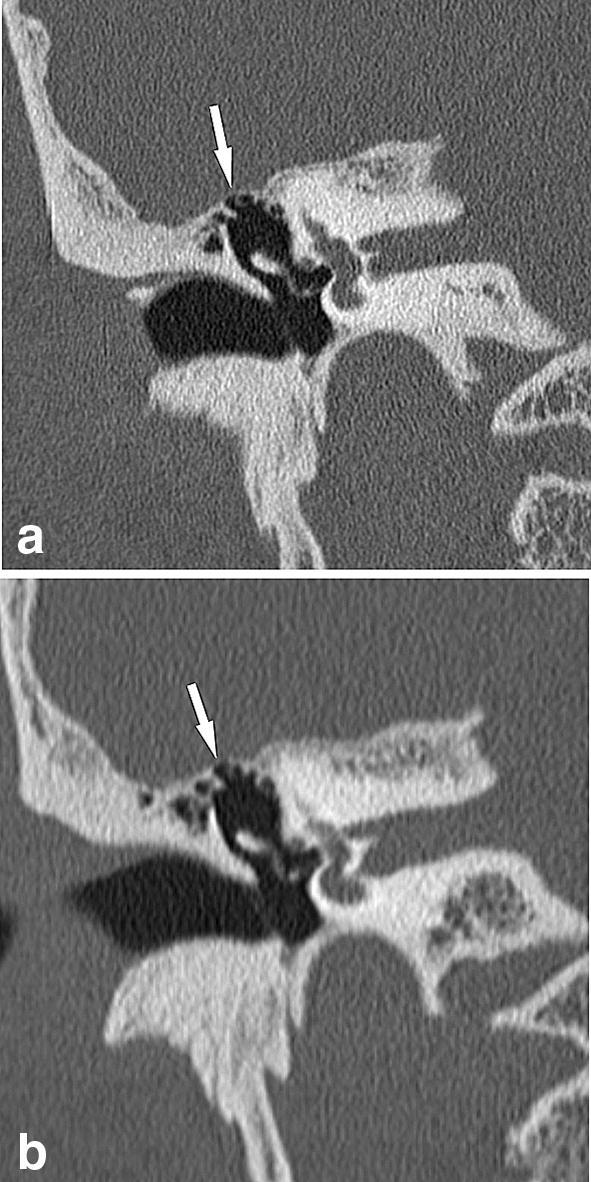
Coronal images of the right temporal bone CT from the same case. Image quality scores for the tympanic tegmen (arrow) are five on UHRCT (a), and three on MDCT (b) by both Reviewers. By both Reviewers, dehiscence in this anatomy was considered to be present on MDCT but not on UHRCT
